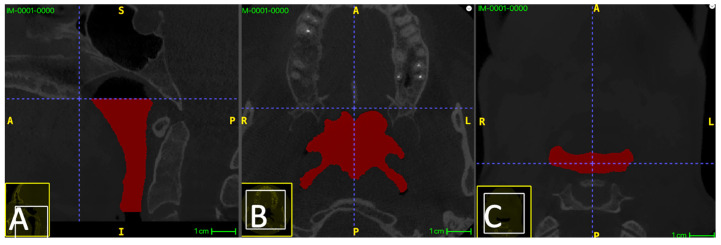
Definition of the segmented upper airway region. (A) Mid-sagittal CBCT view showing the superior boundary plane (Plane S) passing through posterior nasal spine (PNS) and parallel to the palatal plane, and the inferior boundary plane (Plane I) passing through the superior tip of the epiglottis and parallel to the palatal plane. The segmented pharyngeal airway lumen is highlighted in red. (B) Axial slice at the superior boundary (Plane S). (C) Axial slice at the inferior boundary (Plane I).
